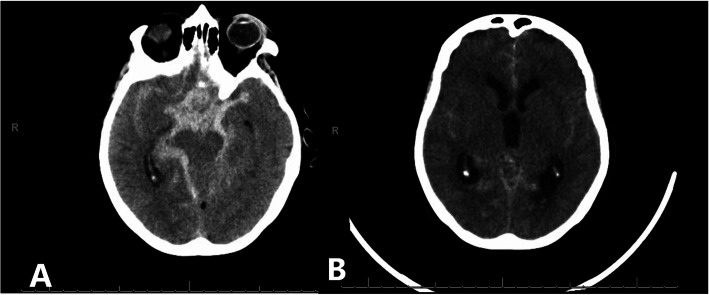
Head CT showing the unique challenges of an aneurysmal subarachnoid hemorrhage patient. a There is a significant amount of basilar cistern subarachnoid hemorrhage placing the patient at high risk for delayed cerebral ischemia. b There is prominent hydrocephalus needing CSF diversion to lower intracranial pressure
